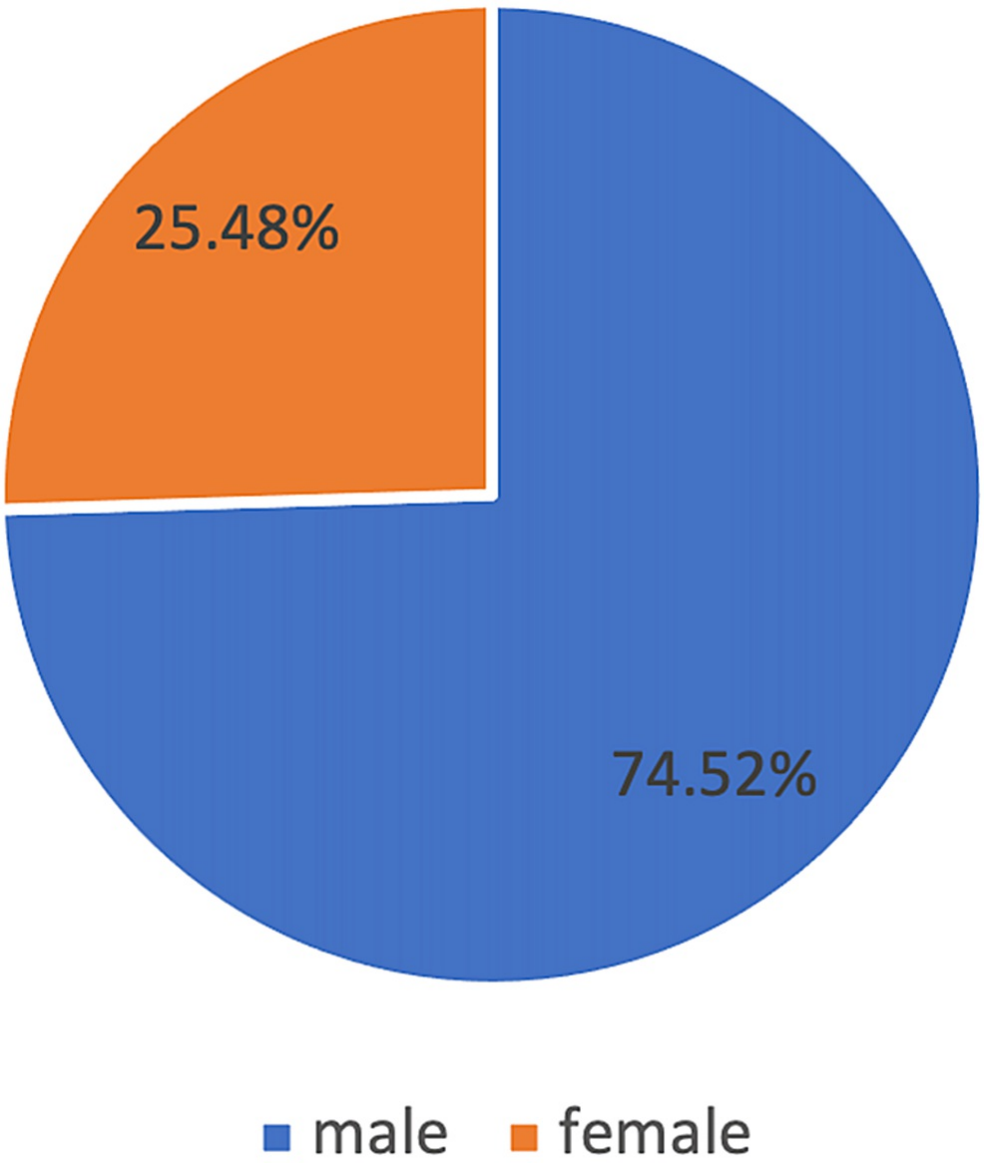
Percentage of male and female patients among the radiographic study sample
